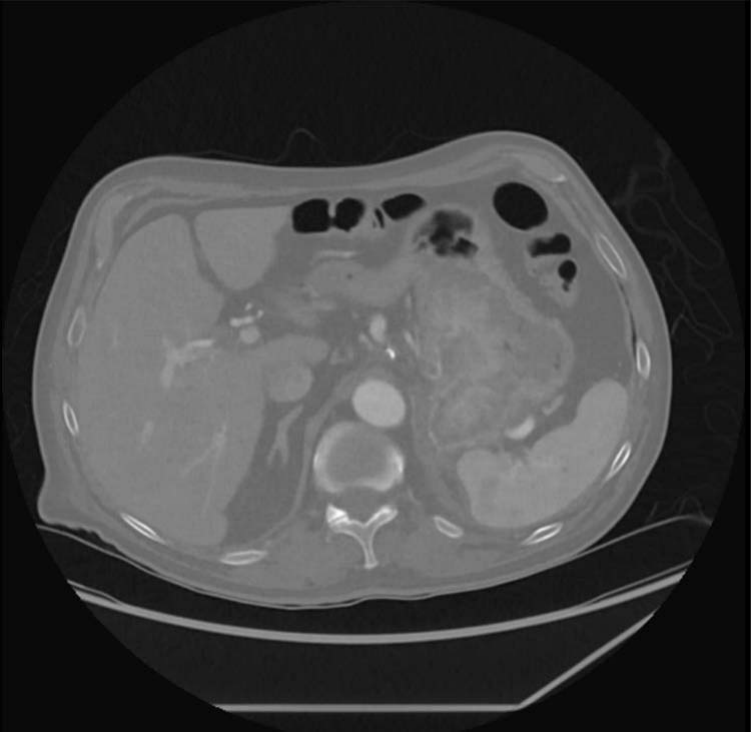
CT abdomen/pelvis with stomach mass.
